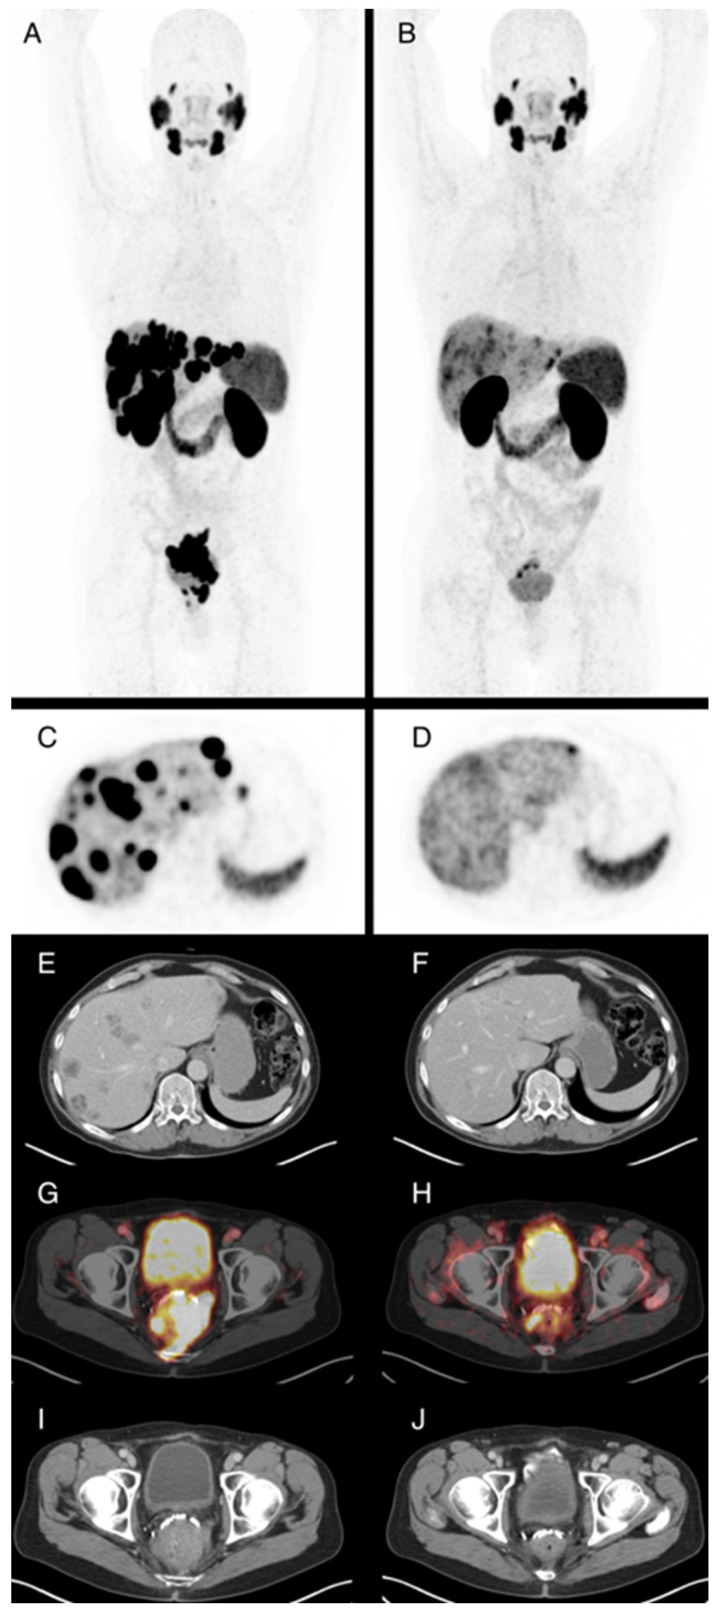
61-year-old patient with locally advanced prostate cancer and metastases in lymph nodes, bone, and liver. Maximum intensity projections of Ga-68-PSMA-PET/CT before (A) and after ((B), SUVmax. 15) four cycles of Lu-177-PSMA radioligand therapy (RLT) with application of 23.6 GBq in total, which demonstrate very good partial response. PSA levels were 25.5 ng/mL prior to RLT and 0.04 ng/mL after the fourth cycle. Axial PET images of the liver before (C) and after (D) therapy show reduction of PSMA-expression of the liver metastases, associated with subtotal reduction of hypodense liver metastases in contrast enhanced CT (E,F). The locally advanced primary cancer also shows substantially reduced PSMA expression of the tumor, with infiltration of the rectum in fusion images (G,H) and contrast enhanced CT images (I,J) from baseline to staging after four cycles of RLT.
